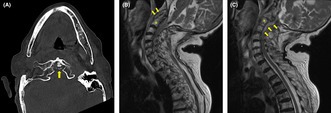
An 88‐year‐old woman with hypertension and chronic kidney disease presented with pain in her left shoulder and neck that had persisted for 2 days. She had no fever, but her pain was exacerbated by forward bending and rotation of the neck. Plain computed tomography revealed calcification of the atlantoaxial joint (Figure 1A). Therefore, she was diagnosed with crowned dens syndrome (CDS) and was prescribed acetaminophen and no antimicrobials. Five days later, she returned because of a fever and difficulty moving. She was hospitalized, and ceftriaxone was administered. Blood culture was positive for *Staphylococcus aureus* the day after admission. Cervical magnetic resonance imaging showed an abscess around the odontoid process (Figure 1B) and an epidural abscess in the spinal canal from C1 to Th2 (Figure 1C). Transthoracic echocardiography showed aortic regurgitation and vegetation adhering to the aortic valve (Figure 2). These observations led to the diagnosis of infective endocarditis.
